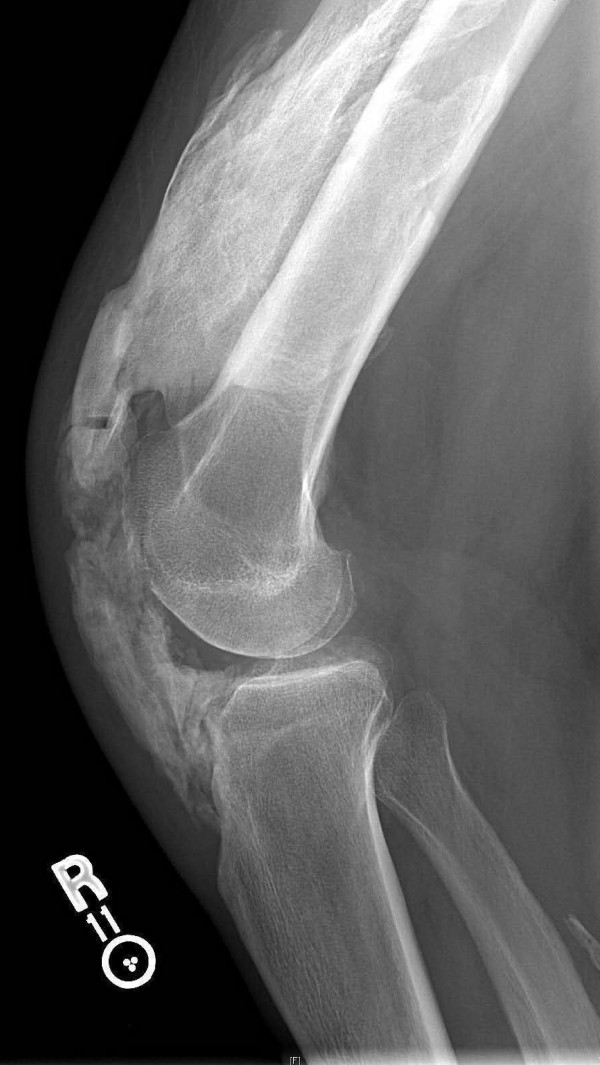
Lateral X-ray three months after patellar tendon repair showing marked progression of HO.
